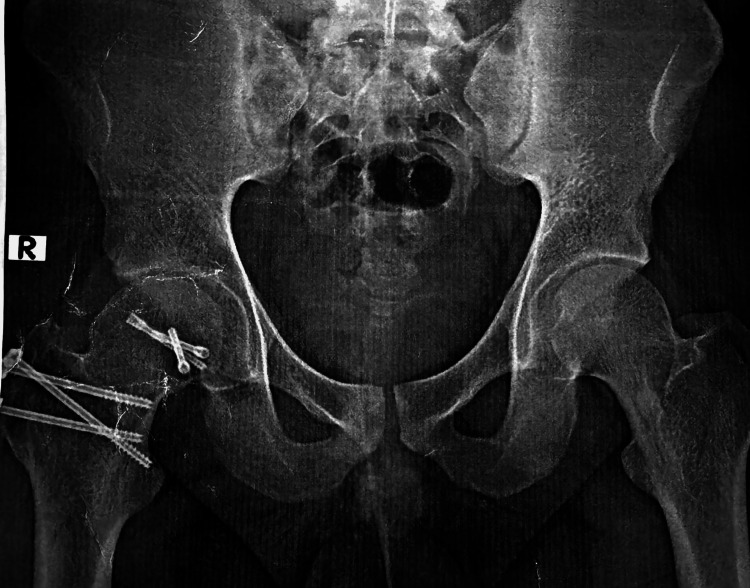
12 months post-operative anteroposterior radiograph of the pelvis with both hips. The radiograph demonstrates complete union of the greater trochanteric osteotomy on the operated right side, maintained reduction of the femoral head fragment with the countersunk headless compression screws in stable position, a concentric and symmetric right hip joint with a preserved joint space, no radiographic evidence of avascular necrosis of the femoral head, joint space narrowing or heterotopic ossification.
